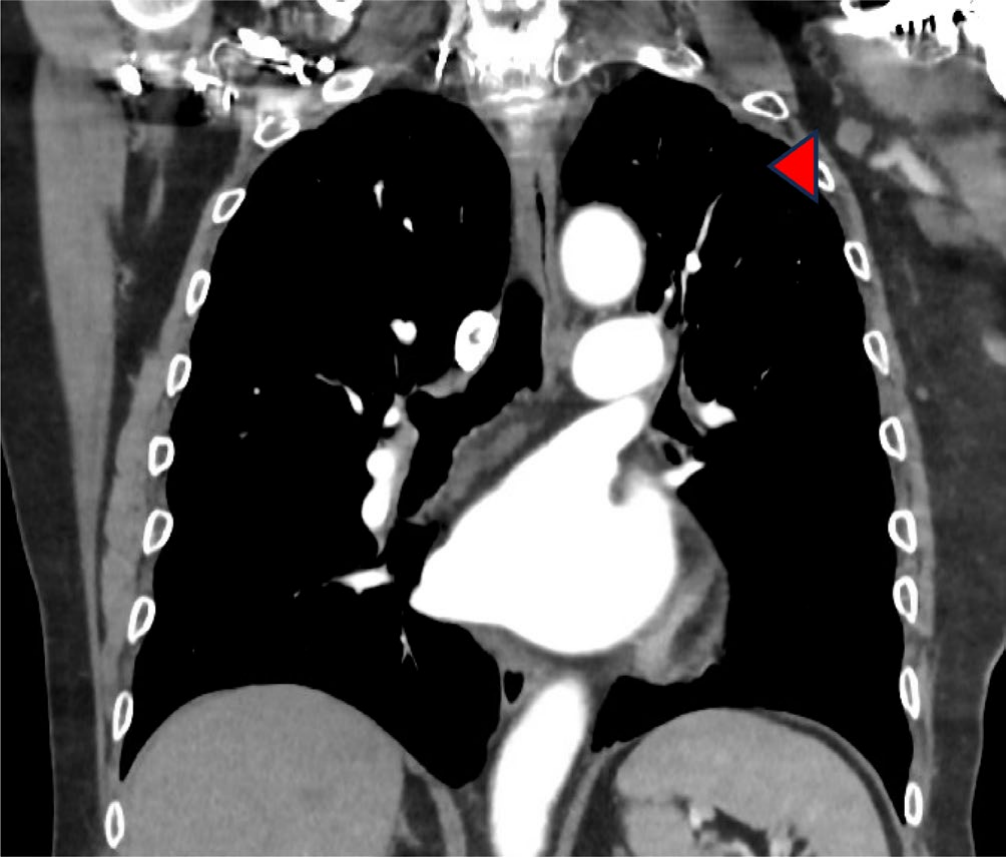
FDG PET‐CT scan showing significant resolution in metastatic lesion (see arrow) in the apical region of the left upper lobe seen in Figure 1 following treatment with pembrolizumab and pazopanib.
